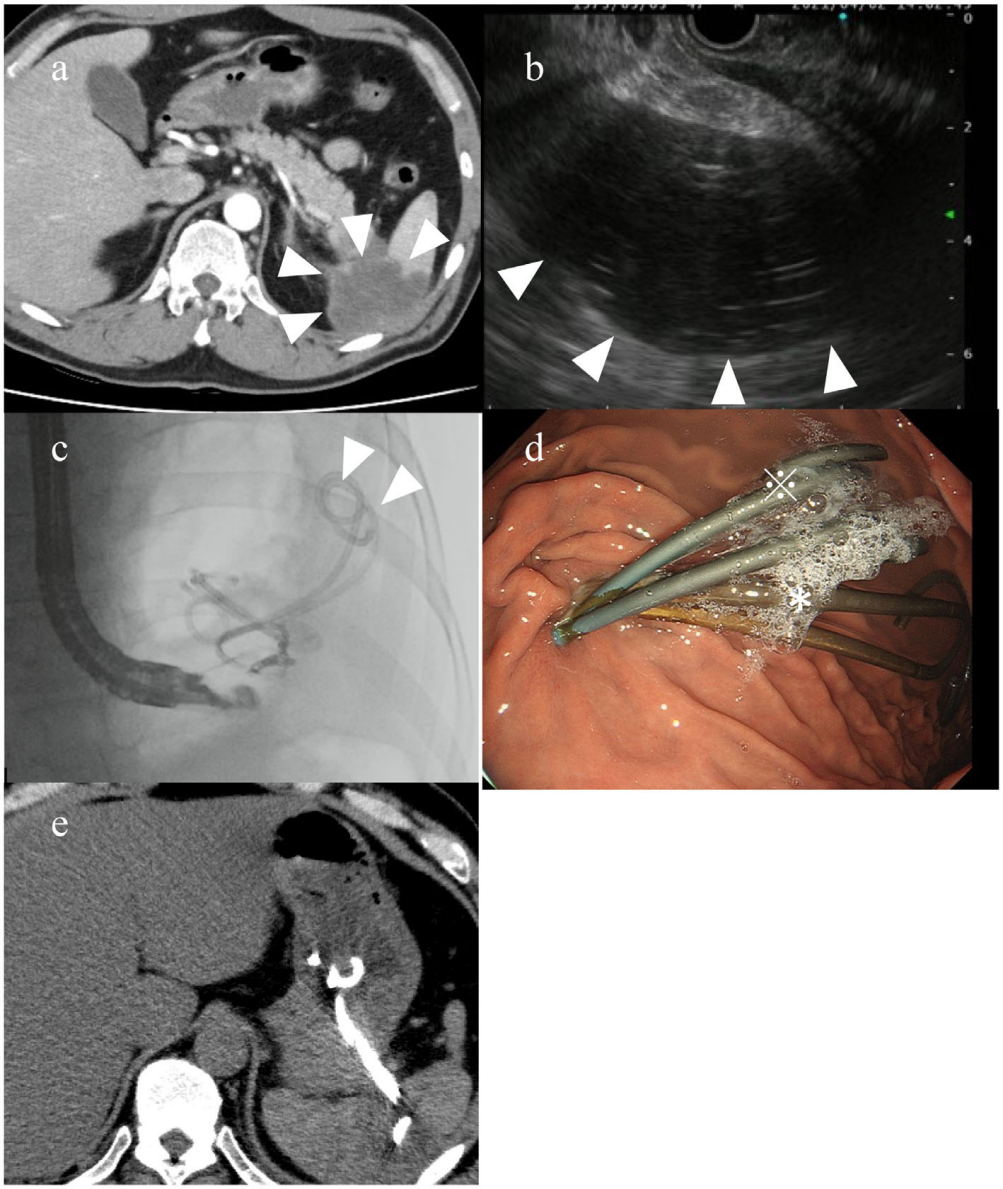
Treatment of a splenic abscess that formed after transcatheter arterial embolization for treating a pseudoaneurysm rupture of the splenic artery. This patient had previously received two plastic stents for draining a pancreatic cyst that formed after acute pancreatitis. (a) Computed tomography (CT) shows a low‐density area in the spleen region, which was considered a splenic abscess (arrowheads). (b) Endoscopic ultrasound image shows a hypoechoic area next to the stomach (arrowheads). (c) We punctured the hypoechoic area from inside the stomach, dilated the fistula with a balloon, and inserted two plastic stents (arrowheads). (d) Endoscopic image inside the stomach shows the two stents inserted for the splenic abscess (*) and two stents that had been inserted previously for a pancreatic cyst (※). (e) CT after EUS‐AD showed two plastic stents from stomach into splenic abscess.
